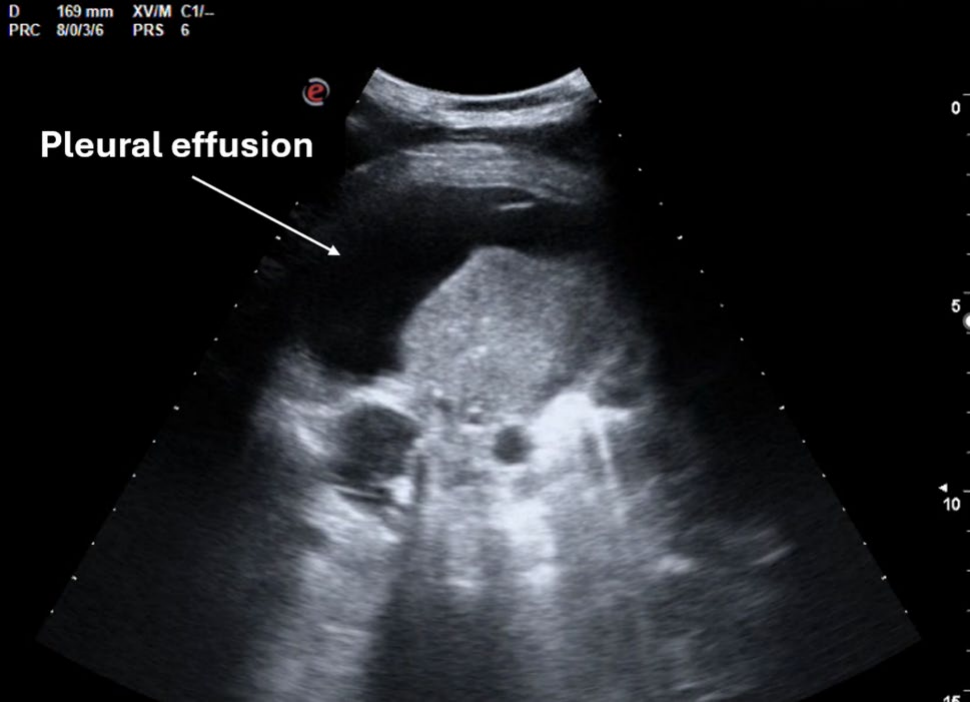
Lung ultrasound (LUS) finding:large pleural effusion
